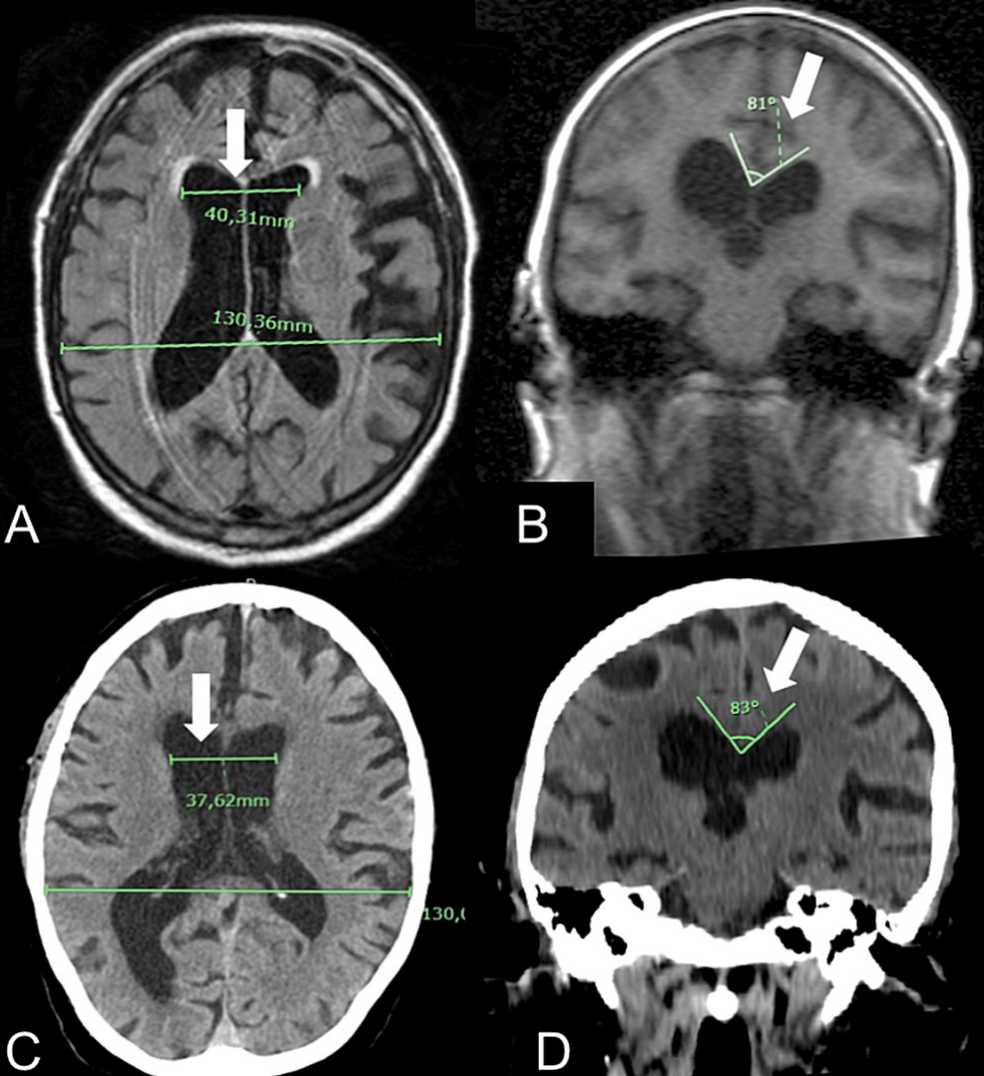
Radiological findings of Case 1 (A, B) Axial and coronal MRI, performed by the patient five months before the hospitalization, showing ventriculomegaly with features of NPH. (C, D) CT scan, performed at the time of neurological deterioration with negative COVID test, showing no substantial modifications of the ventricular system volume. The white arrows highlight the common features of NPH: Evans Index (A, C)>0.28; 0.31; callosal angle (B, D) < 100°.
